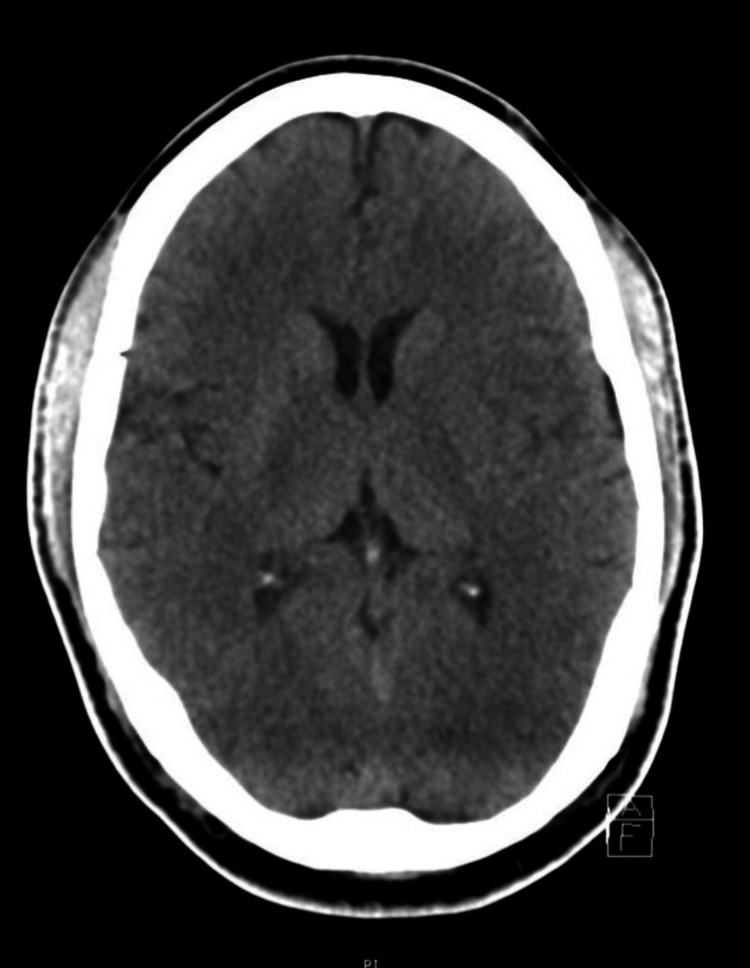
Computed tomography (axial view), unremarkable study
